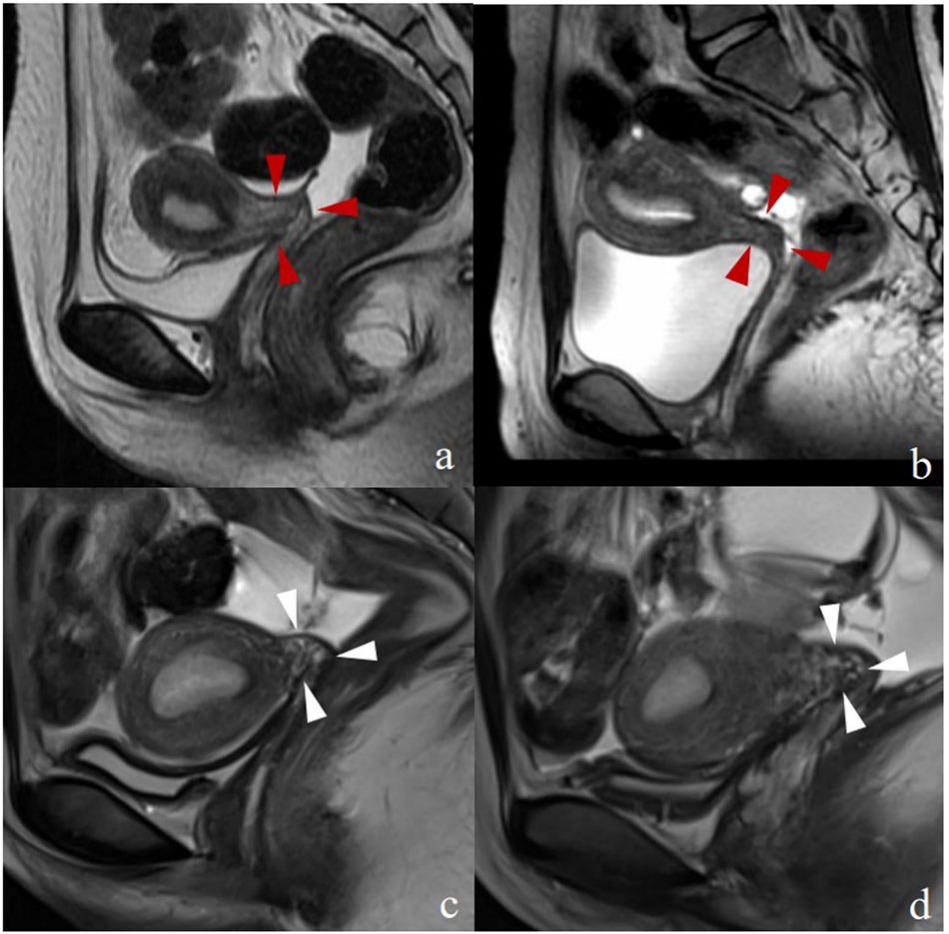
Magnetic resonance images depicting four individuals classified as having type II congenital cervical malformations. The sagittal T2-weighted images of these patients revealed the absence of luminal structures. Among these, two displayed homogeneous signals (red arrowheads in a and b), while the remaining two exhibited conspicuous mixed signals (white arrowheads in c and d)
